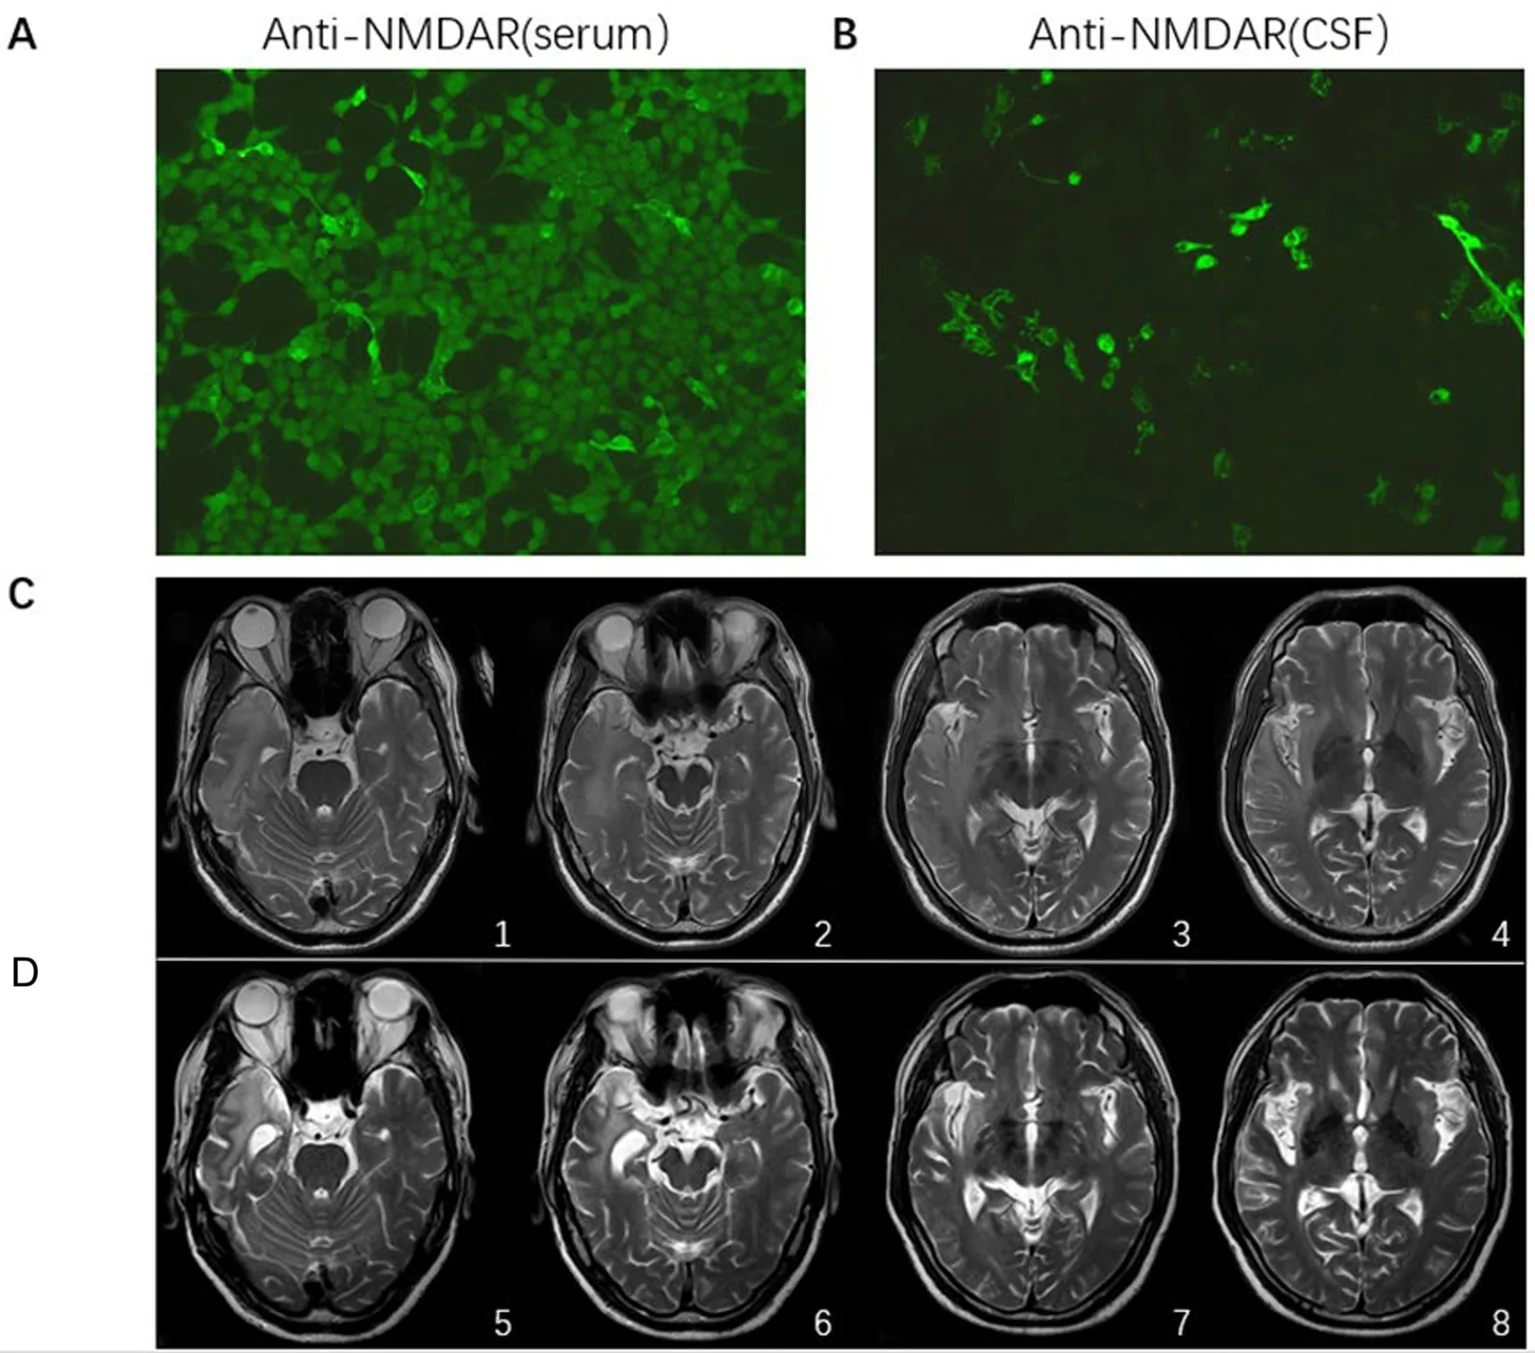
NMDAR antibody and cranial MRI changes of patient no. 4 (A,B) show serum and CSF positivity for NMDAR antibody. (C) 1–4 Show lesions with abnormal signals in the right frontoparietal lobe, bilateral temporal insulae, and right thalamus on FLAIR sequences before EFG treatment. (D) 5–8 Display reduction in lesion size and concomitant alleviation of perilesional edema post-EFG. FLAIR, fluid-attenuated inversion recovery; NMDAR, N-methyl-D-aspartate-receptor.
